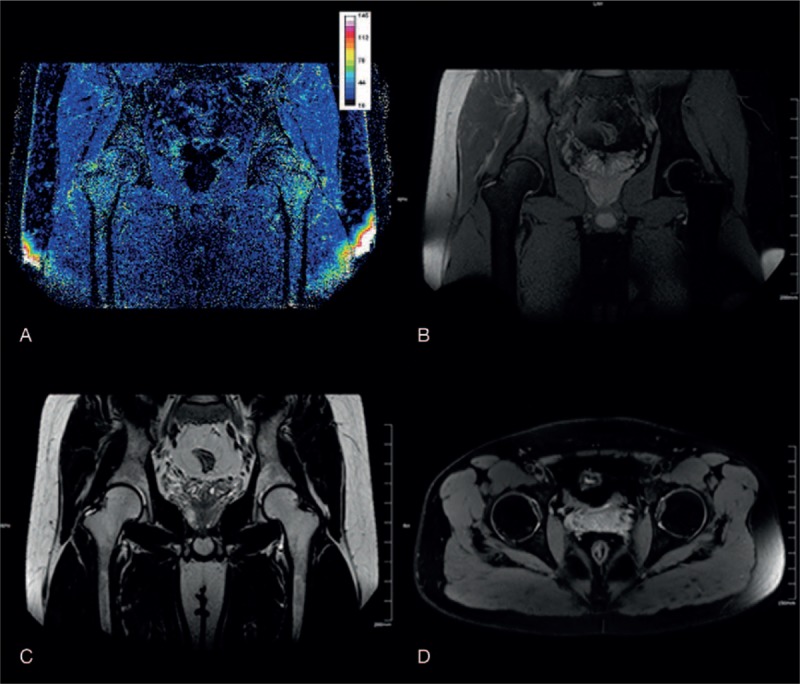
The BOLD-MRI mapping and conventional MR image of a healthy volunteer. (A) R2∗ mapping reflected the structure and blood supply variation of the femoral head. The values of zones 4 and 1 were highest, followed by zones 6 and 3, and zones 5 and 2 had the lowest values. Conventional MRI (B–D, coronal PDW/SPAIR, coronal T2WI/TSE, axial T2WI/SPAIR) showed a normal femoral head without any disease. BOLD-MRI = blood oxygen level-dependent MRI, R2∗ = total transverse relaxation time, TSE = turbo spin echo.
